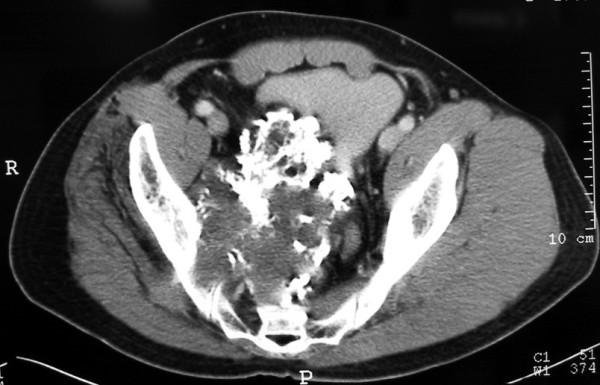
CT scan of the pelvis showing the tumor sized 12 cm × 10 cm originates from the right pelvis and invades the sacroiliac joint and pelvic cavity.
